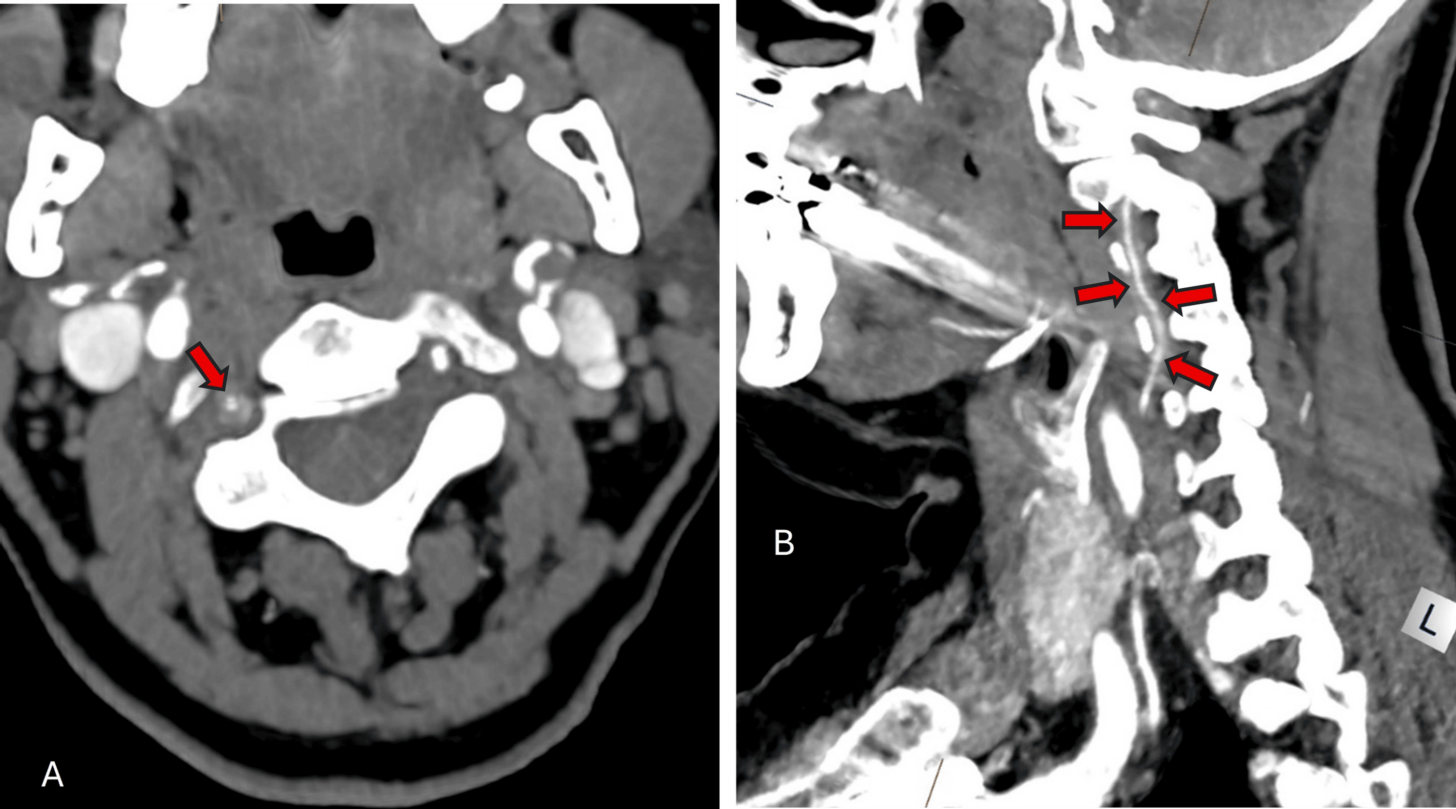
Axial and sagittal section of a CT angiogram Image A is an axial section of a CT angiogram and shows narrowing of the right vertebral artery. The red arrow indicates possible intramural thickening. Image B is a sagittal section of the left vertebral artery and its cervical course. Although difficult to appreciate, the red arrows are pointing towards the wall of the artery and again suggest that there may also be intramural thickening.
